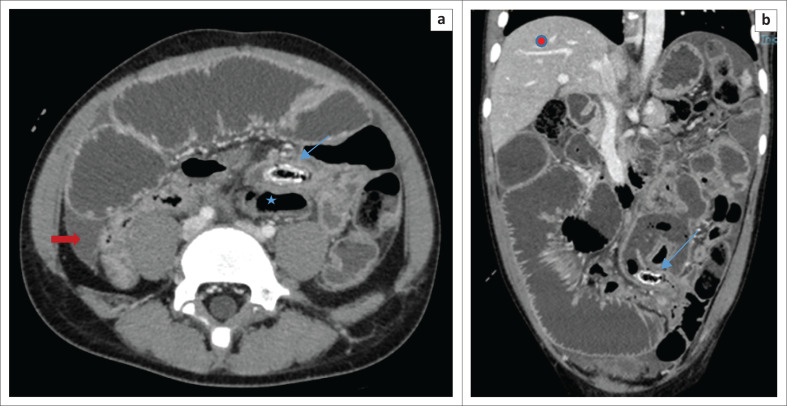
(a and b) Axial and coronal CT images demonstrating appendiceal wall thickening and enhancement with a faecolith (blue arrows) anterior and cephalad to the caecum (star). The right iliac fossa was unremarkable with fluid in the paracolic gutter (red arrow). Note the liver (red circle) is in the normal anatomic position.
